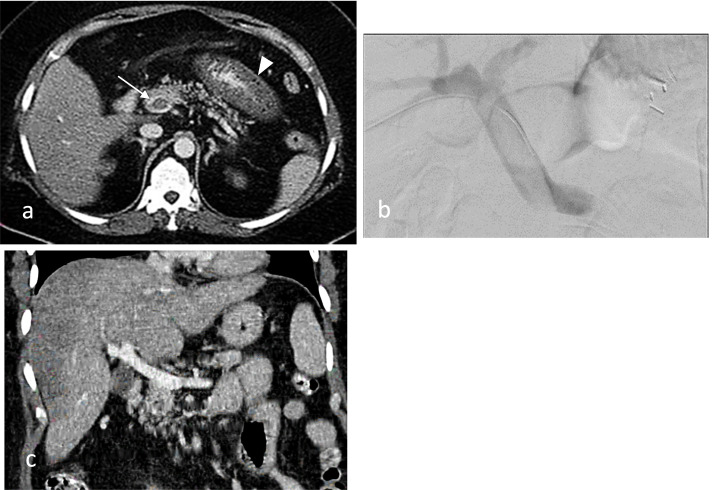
a Pre-procedural Contrast-Enhanced Computed Tomography (CECT) showing portal vein thrombosis (arrow) and jejunal wall thickening (arrow-head). b Pre-procedural trans-hepatic portogram showing main portal vein thrombosis with patency of intrahepatic portal branches. c Follow-up Contrast-Enhanced Computed Tomography (CECT) demonstrating recanalization of the portal vein
